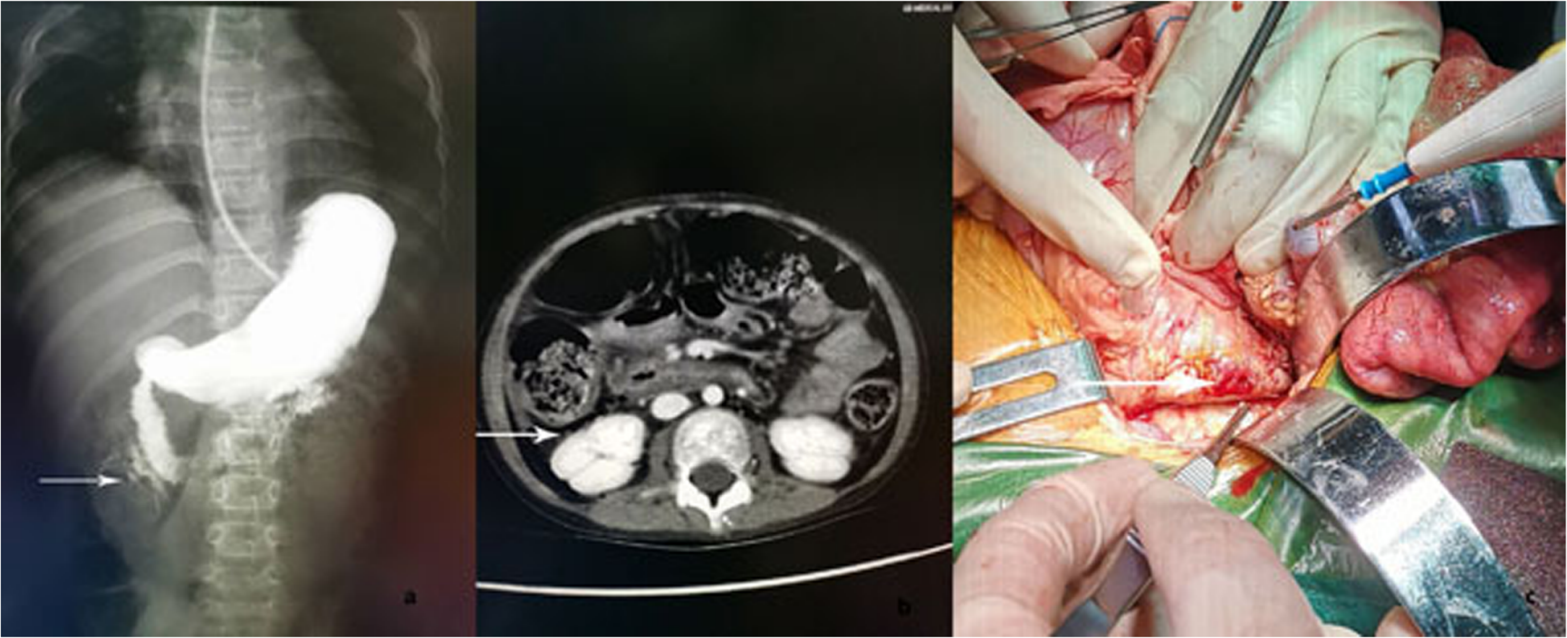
a Contrast leakage seen at the junction of the descending and horizontal segments of the duodenum on Case 4 (arrow). b Contrast-enhanced CT showed retroperitoneal gas on Case 4 (arrow). c Duodenal perforation can be seen at surgery on Case 4 (arrow)
